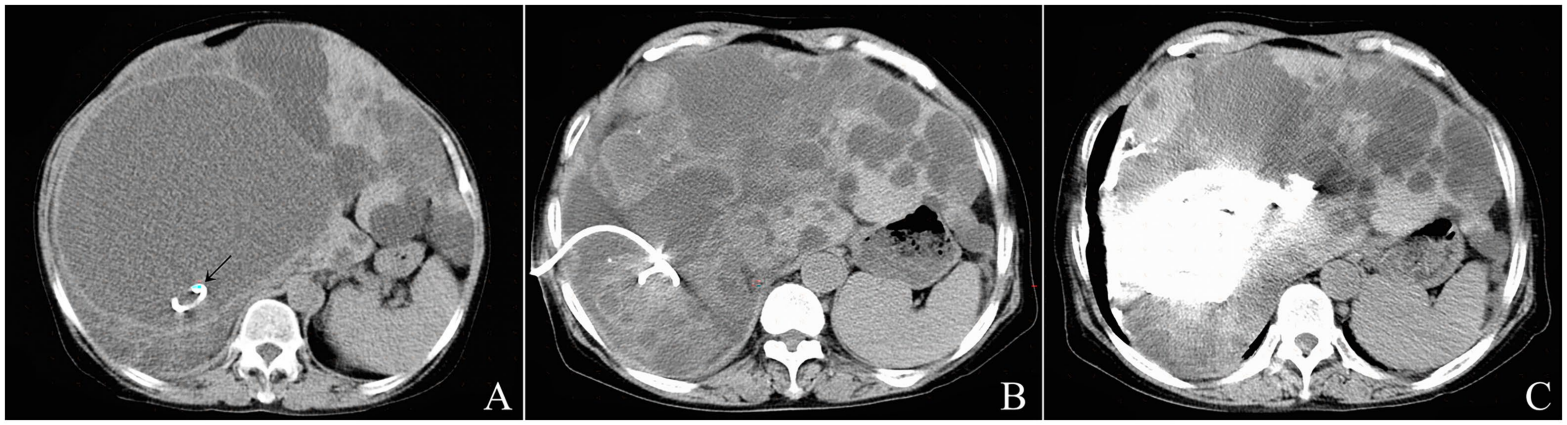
The operation procedure of CT-guided percutaneous catheter aspiration and bleomycin sclerotherapy for a 64-year-old female patient with a giant liver cyst in polycystic liver disease. After inserting percutaneously a 8.5-Fr drainage catheter into the acyst cavity using the Seldinger technique, the position of the tip of central venous catheter (arrowhead) was confirmed by the control CT scan (A). A recheck CT scan on the next day showed complete evacuation of the cyst (B). After injection of bleomycin solution and removal of pigtail catheter, postprocedure CT scan showed nonoccurrence of sclerosant extravasation (C).
